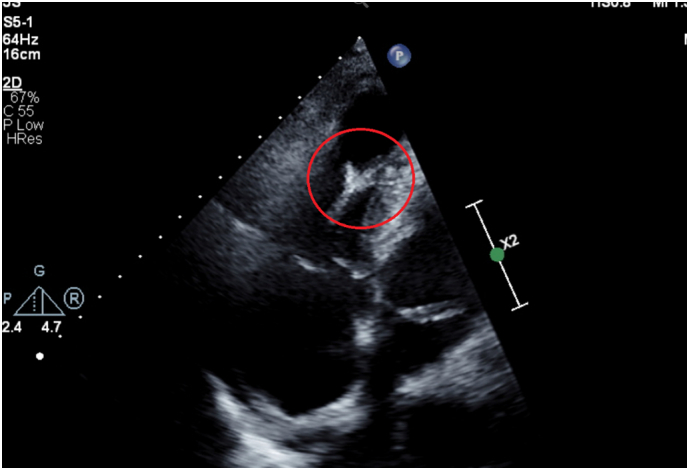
Apical four chamber view demonstrating partially avulsed papillary muscle.
